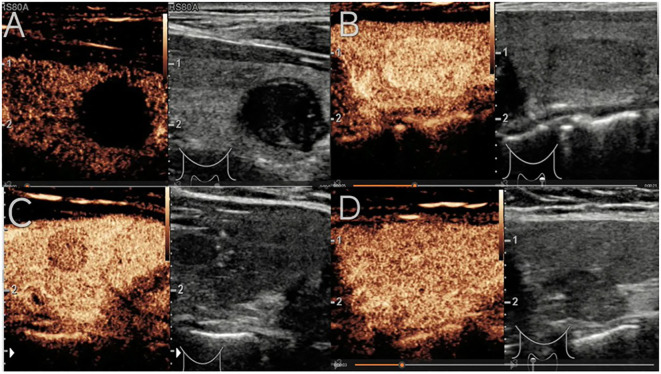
Contrast-enhanced ultrasound (CEUS) image (left) and conventional ultrasound image (right). (A) Non-enhancing nodule with benign FNA pathology; non-enhancement is a highly specific benign marker. (B) Homogeneous hyperenhancement, benign nodule. (C) Hypoenhancement confirmed papillary thyroid carcinoma (PTC) by surgery. (D) Isoenhancement, benign nodule. Abbreviations: CEUS, contrast-enhanced ultrasound; FNA, fine-needle aspiration; PTC, papillary thyroid carcinoma.
